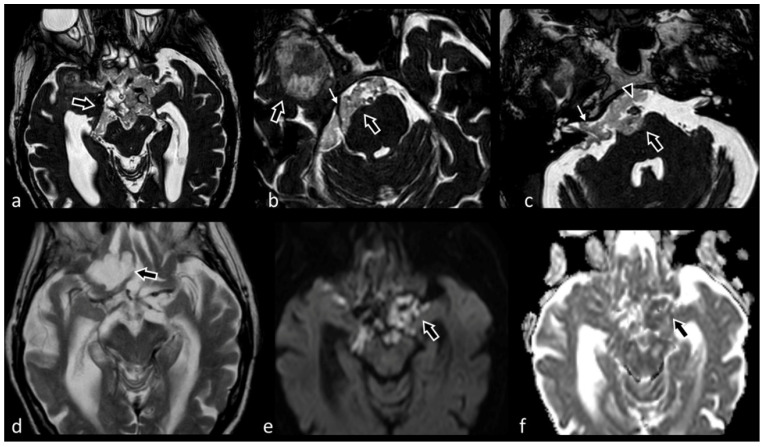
Voluminous extra-axial lesion centered in the suprasellar cistern, diffusively extending into the contiguous cisternal spaces as well as into Sylvian and choroid fissures bilaterally; inferiorly, the mass also runs along the prepontine cistern, the Meckel’s cave and the internal auditory canal (small white arrow in (c). The lesion appears isointense to CSF in T2-TSE (d), markedly hyperintense on DWI (e) and partially and lightly hypointense on ADC maps (f). 3D CISS (a–c) is superior compared to the other sequences in demonstrating the internal texture of the lesions, its margins as well as its relation with surrounding structures (e.g., fifth cranial nerve, small white arrow in (b), and basilar artery, arrowhead in (c), which are encased but not dislocated by the mass). These features, alongside the characteristic hyperintensity in DWI, are highly suggestive for an epidermoid cyst.
